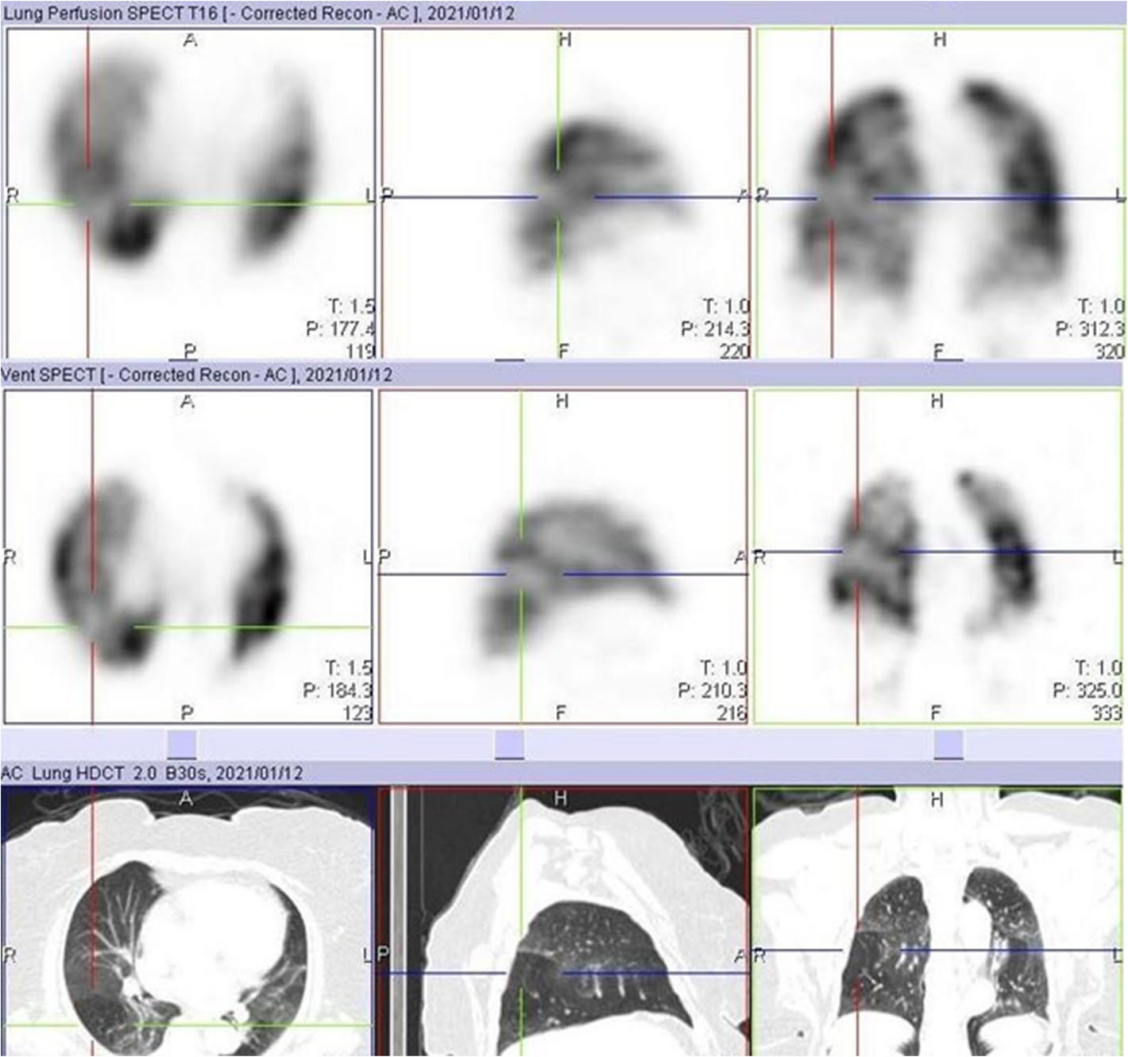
Ventilation perfusion SPECT/CT study of the same patient in Fig. 3, performed 3 months after her initial perfusion only SPECT/CT study. The previously seen defect on the initial perfusion only study in the right lung (crosshair) is matched
